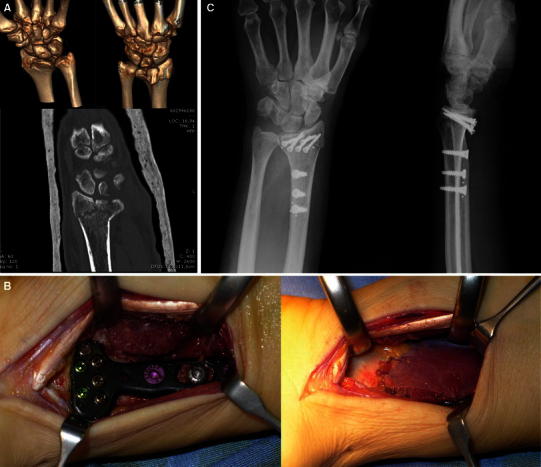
a Preoperative 2- and 3-D CT scan showing a C1-type wrist fracture according to the AO classification. b Intraoperative view of distal radius fracture with the DiPHOS-RM implanted and the pronator quadratus re-attached. c X-ray evaluation performed 3 months after surgery showing good healing of the fracture
